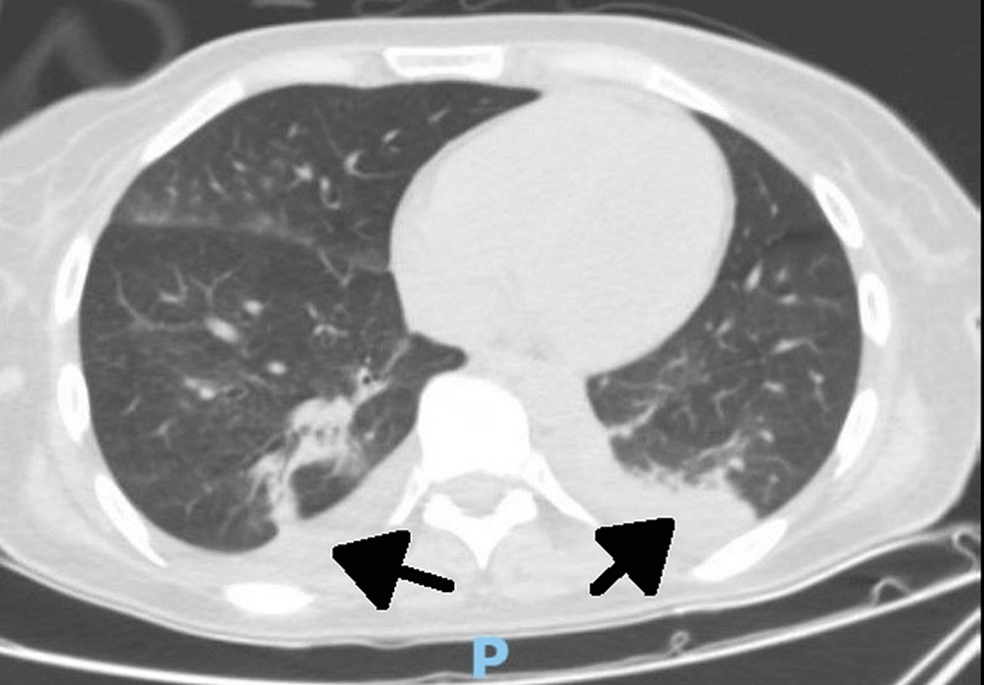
Computed tomography scan of the thorax showing bilateral pleural effusion (arrows).
